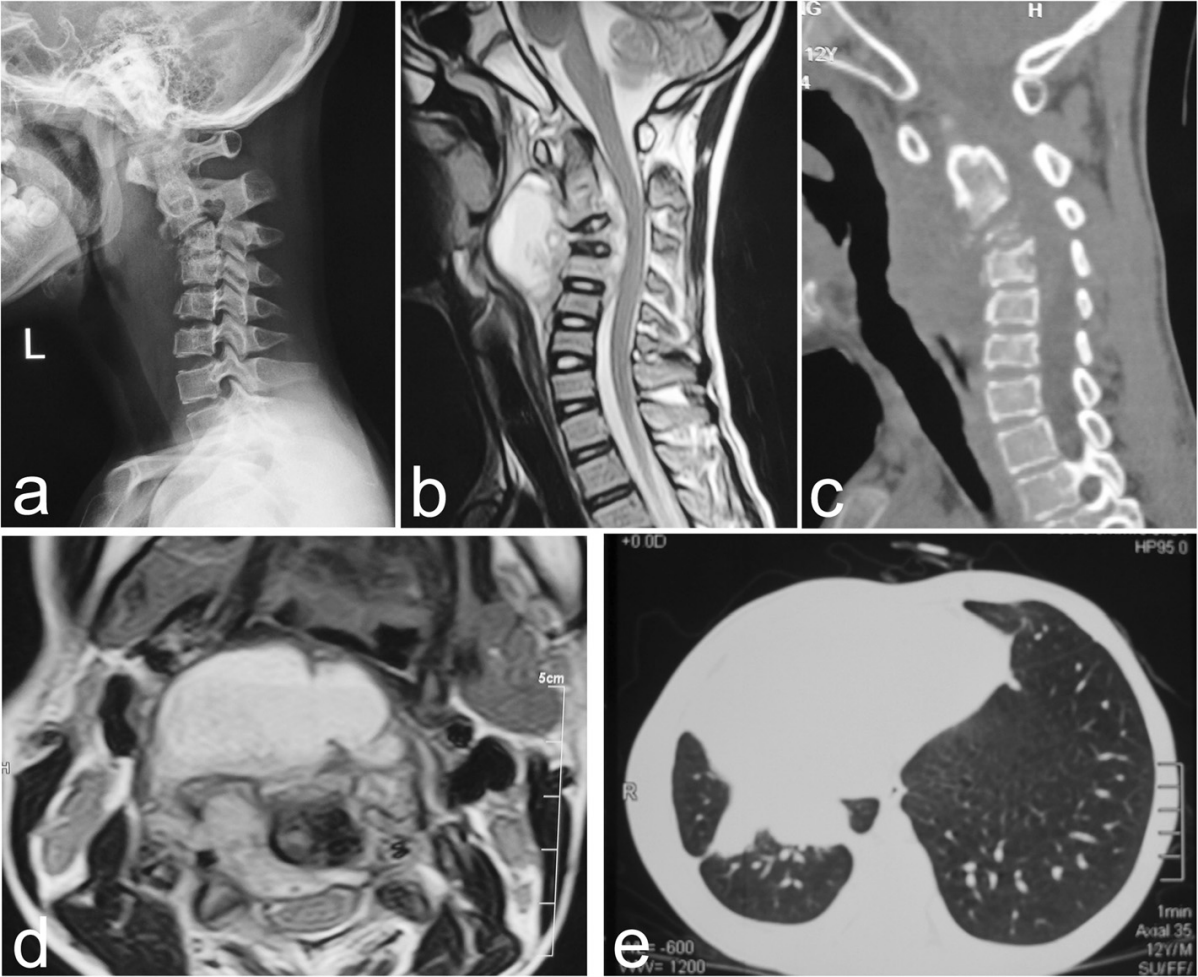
Preoperative images of patient no. 5. (a) Lateral X-ray of a 12-year-old male child demonstrated vertebral body damage and sagittal instability and kyphosis. A preoperative sagittal MRI (b) and CT (c) revealed significant C3-4 vertebral body destruction with kyphosis associated with epidural, paravertebral and retropharyngeal abscess formation; and the cervical spinal cord was severely compressed. Coronary MRI (d) revealed a huge abscess that affected the esophagus and invaded into the spinal canal. (e) CT scan and enhancements of the lung: the right thorax collapsed and pleural thickening occurred, suggesting tuberculous pleurisy and encapsulated pleural effusion
